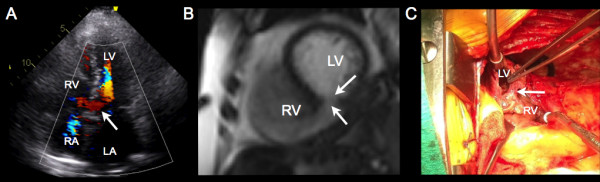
A) An apical 4 chamber view on TTE demonstrating left to right shunting across the interventricular septum on color Doppler (arrow); B) Short axis balanced steady-state free precession CMR image demonstrates rupture of the mid inferior septal segment (arrows) with free communication between the right (RV) and left (LV) ventricles; C) A trans-ventricular exposure of the diaphragmatic surface through the infarcted myocardium at the time of surgery. The infarct incision on the diaphragmatic surface of the heart reveals the VSR, with a free edge (arrow) that has already matured. RA, right atrium; LA, left atrium; RV, right ventricle; LV, left ventricle.
